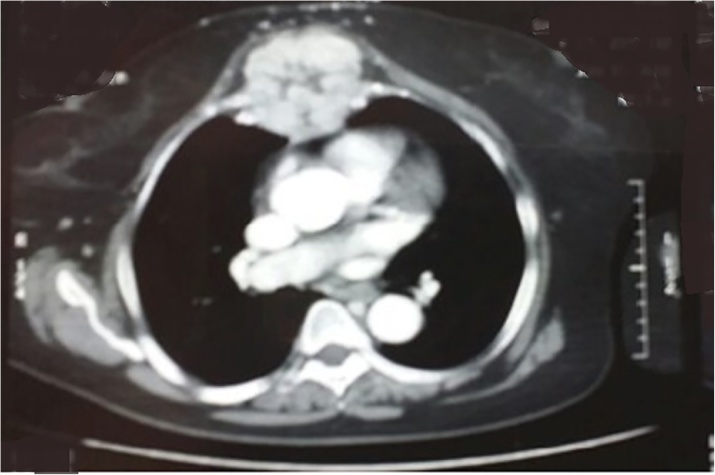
CT scan of chest showing a mediastinal mass.
